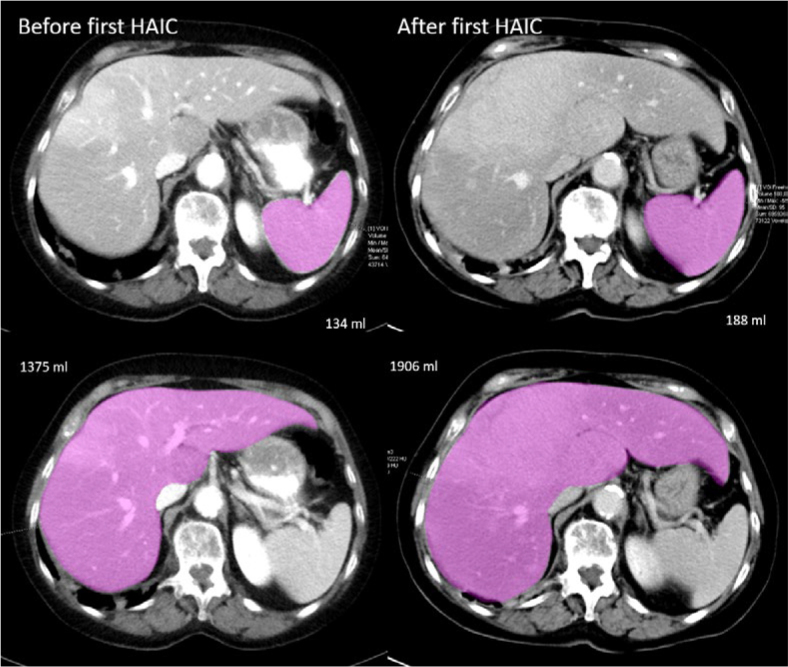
71-year old women with uveal melanoma and liver metastases. Axial CT image examples of volumetry of liver and spleen four days before and 44 days after first hepatic artery infusion therapy (HAIC). Liver and spleen volume increased by 39% after first HAIC.
